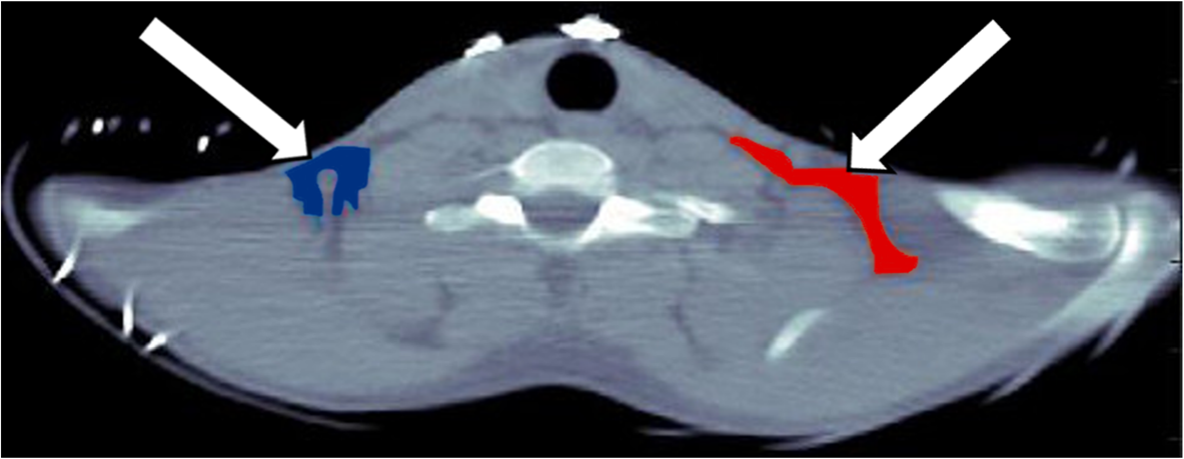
Typical regions of interest placement for brown adipose tissue based on axial CT images. These regions of interest cover the whole supraclavicular fat depot
